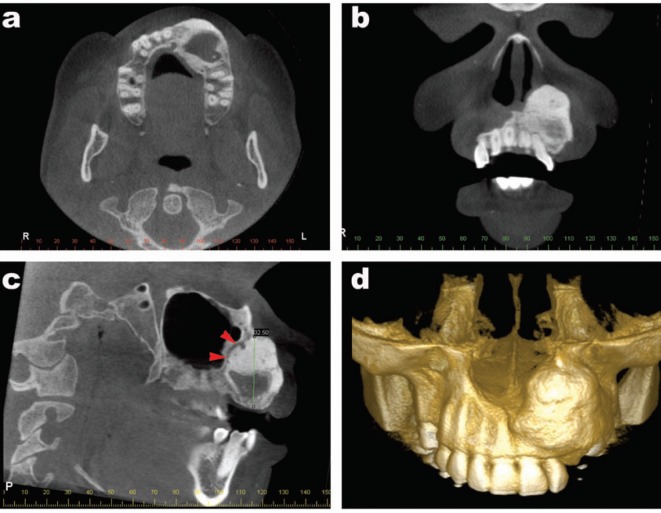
Cone beam computed tomography (CBCT) features of ossifying variant of odontogenic fibroma. a) Axial plane showing a mixed hyperdense-hypodense image with buccal expansion in the left maxilla. b) Coronal plane showing root resorption of teeth 21 and 22. c) In the parasagital plane, a mixed image measuring 32.5 mm associated with a hypodense line (red arrows red) delimiting the lesion of the health bone. d) 3D-CBCT showing buccal expansion of the lesion associated with invasion of the nasal cavity.
